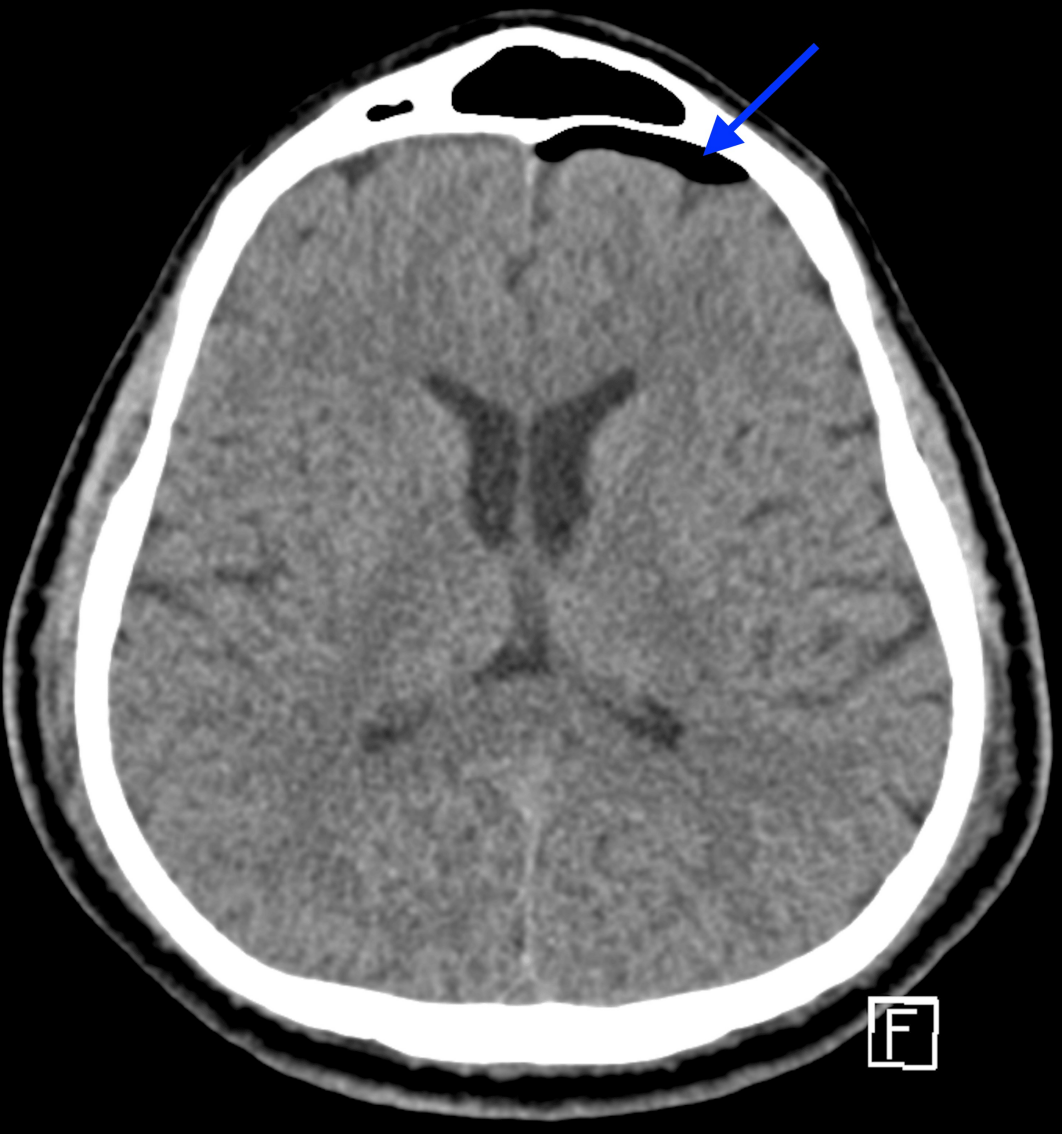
Axial CT head without contrast on postoperative day 2 - image 1 The image demonstrates a new left frontal pneumocephalus (arrow), suggesting CSF leak with air entry CSF: cerebrospinal fluid; CT: computed tomography
